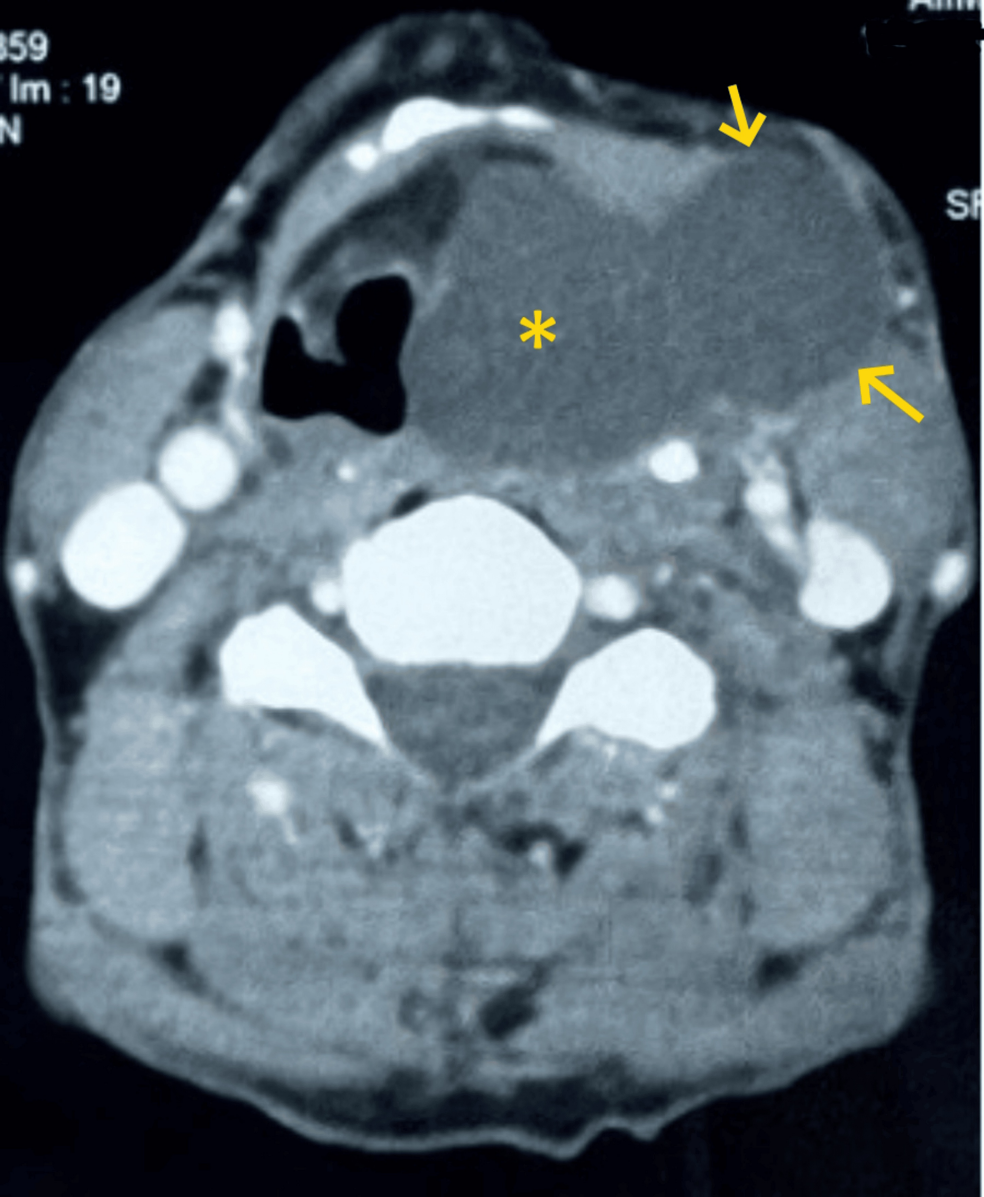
Contrast-enhanced computed tomography (CECT) of the neck showing a cystic lesion in the left level III cervical region, extending from the left anterior visceral space (*) into the left anterior cervical space (arrows). The lesion displaces the epiglottis and the left aryepiglottic fold toward the contralateral side.
